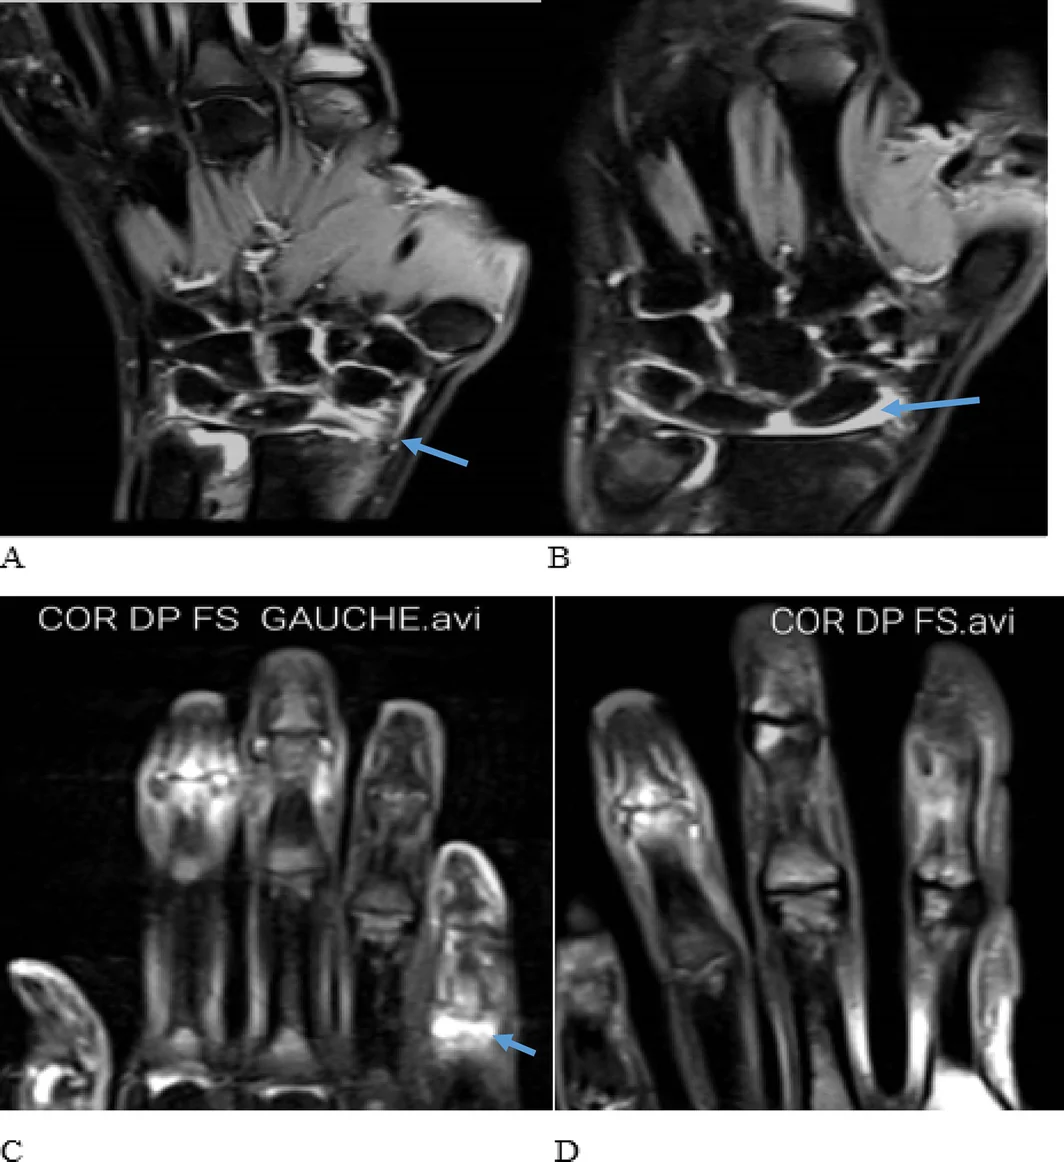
MRI of the hands and wrists using DP FAT‐SAT sequences showing bone erosions of the distal end of the radius (A, arrow), intra‐articular radiocarpal effusion (B, arrow), and erosions with intra‐articular effusion of the DIP joints (C, arrow; D), consistent with synovitis.
